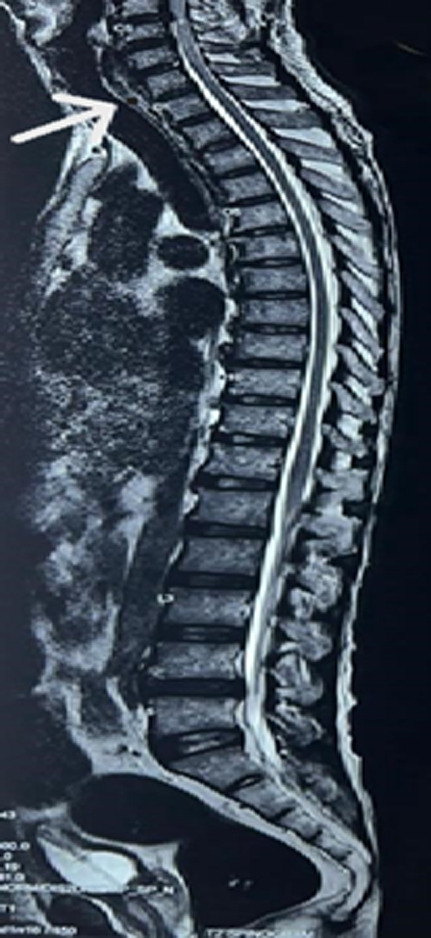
magnetic resonance imaging of the cervical spine with contrast and screening of dorsolumbar spine showing subcentrimetric tarlov cyst at neural foramina aspect of C7/D1 level
